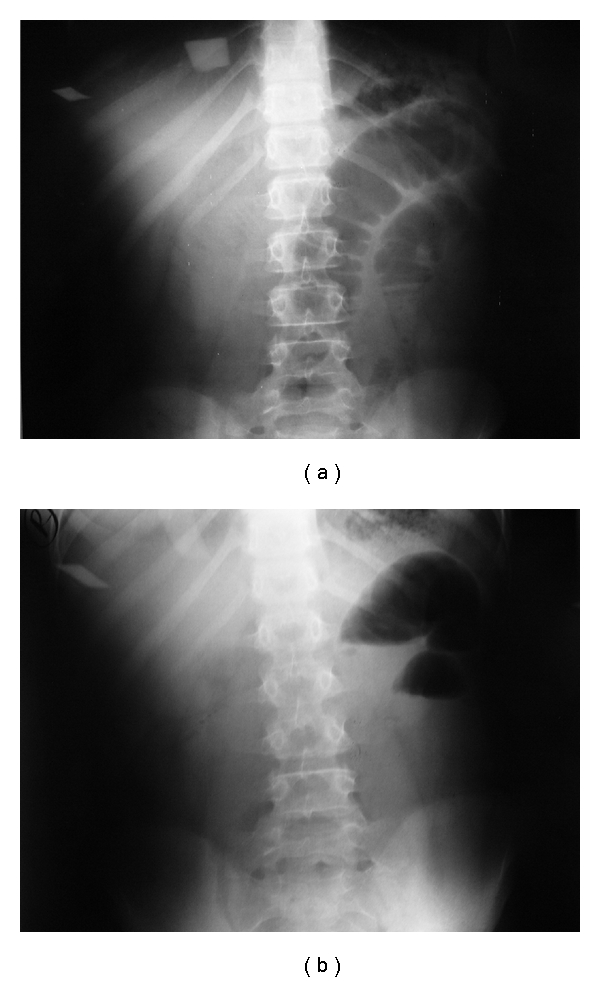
Plain abdominal X-rays showing dilated loops with multiple air fluid levels.
